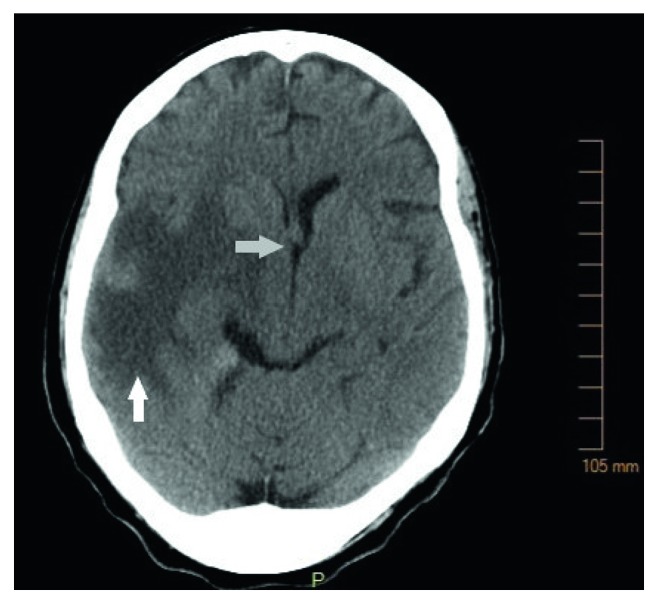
Computed tomography (CT) head imaging demonstrating a large focus of edema involving the right temporal lobe (white arrow) as well as hyperdense dural thickening in the right medial petrous apex with 6 mm of midline shift (grey arrow).
